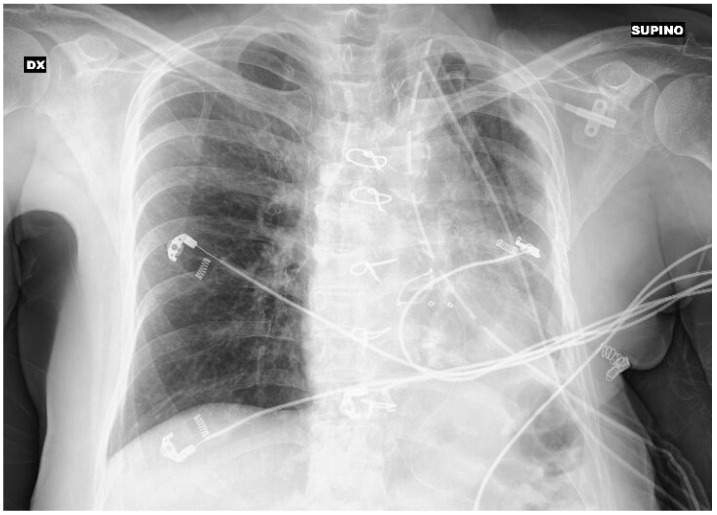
Chest X-ray after surgical revision.
